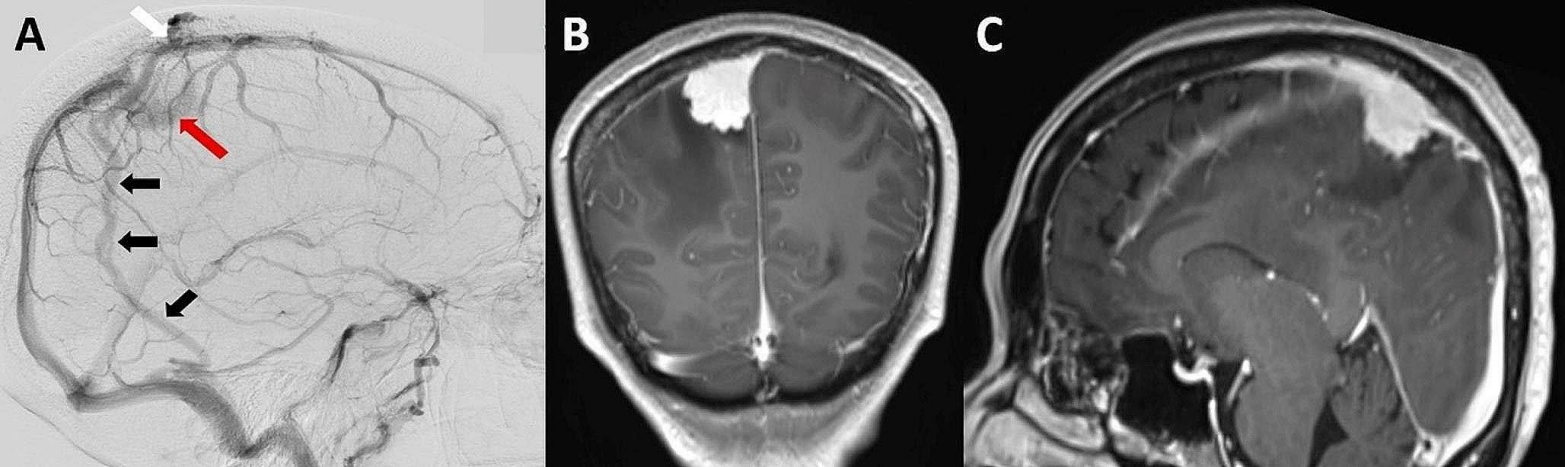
CVP with high risk of inadvertent injury during craniotomy. DSA and contrast-enhanced cranial MRI in case no.9 (A-C). (A) DSA showing an OP route of DV (black arrows) as the main CVP. The origin of the OP route of DV in the SSS is labeled as a white arrow, and the course of the OP route of DV is very close to the blushing of the tumor (red arrow); and (B, C) contrast-enhanced cranial MRI in coronal and sagittal views, pathological diagnosis was meningioma. CVP, collateral venous pathway; DSA, digital subtraction angiography; DV, diploic vein; OP, occipitoparietal route; SSS, superior sagittal sinus
